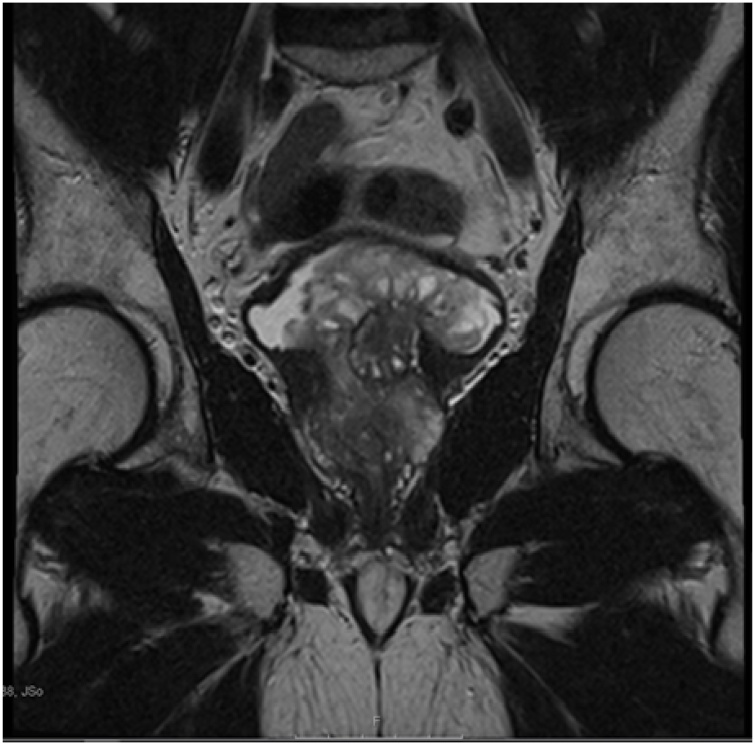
T2-weighted MRI scan (coronal view) shows the regrowth of the prostate gland into 131 ml protruding to the bladder base after only one year of TURP.
